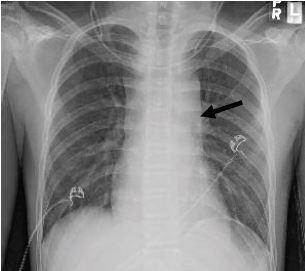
Upright chest radiograph showing a widened mediastinum (arrow) in a 12-year-old with vascular Ehlers-Danlos Syndrome.
